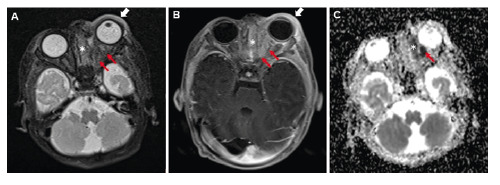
Axial section T2-weighted brain magnetic resonance imaging (MRI) (A) demonstrates diffuse intensity increases indicative of sinusitis in the ethmoid cells and medial rectus muscle. Furthermore, an increase in intensity and thickness is evident in the left periorbital area. Axial section T1-weighted brain MRI with contrast (B) reveals increased contrast enhancement in the left extraocular muscles, particularly noticeable in the rectus medialis and the intraconal-extraconal fatty planes. The apparent diffusion coefficient image (C) shows a hypointense signal, which indicates restricted diffusion in the ethmoid cells and the medial rectus muscle. (Ethmoid cells; asterisk, white arrow; periorbital area, red arrows; medial rectus muscle).
